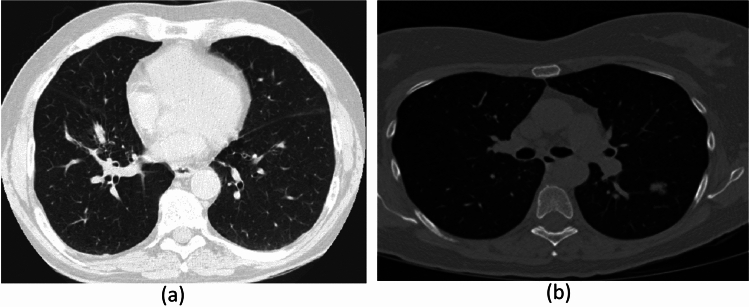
sample of dataset CT image Kaggle Bowl2017, (a) normal, (b) abnormal.
